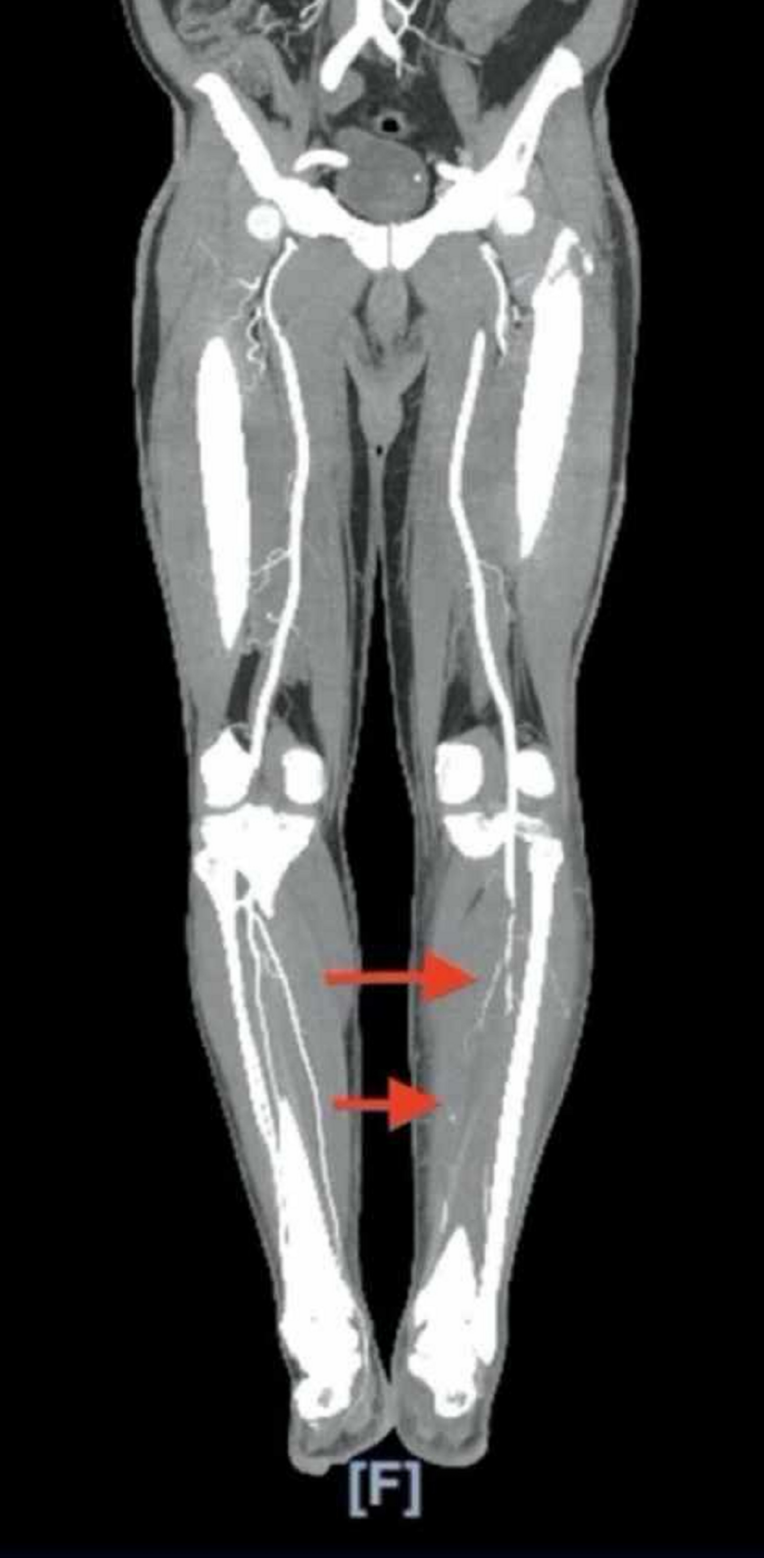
CT angiography of aorta with iliofemoral runoff showing occluded left anterior tibial artery and left peroneal artery.
